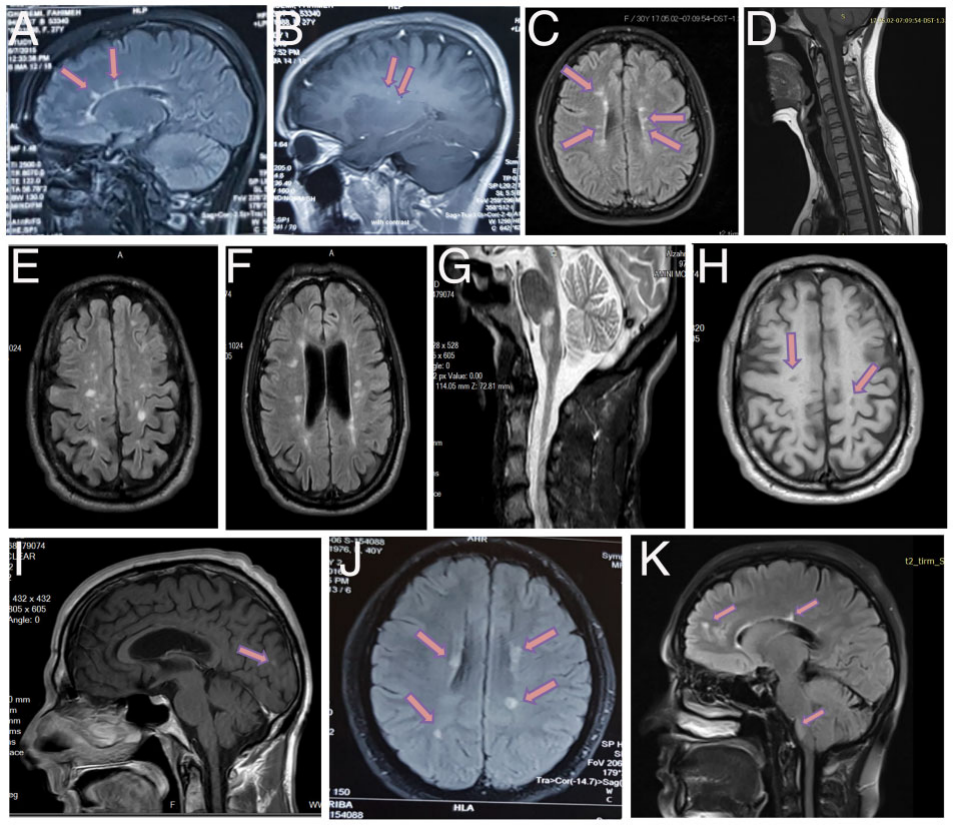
Brain MRI. Axial contrast-enhanced T2W MRI image of the brain of Cases V:6, V:1 and V:3. *Case V:6* (A) Midline sagittal flair (B) and parasagittal T1+GAD images, at 27 years of age, show multiple high-signal foci in the corpus callosum and paraventricular space with enhancing. (C) Axial FLAIR image, at 29 years of age, shows bilateral periventricular high-signal foci. (D) Spinal cord atrophy is shown. *Case V:3* (E, F) Axial FLAIR images, at the age of 39, show bilateral juxta cortical, periventricular and paraventricular high-signal foci and ventricular dilation due to brain atrophy. (G) Sagittal T2 weighted image shows confluent plaques in pons and medulla. (H) Axial T1 weighted image shows some black hole lesions in paraventricular space due to severe axonal injury and loss. (I) Faint enhancement after contrast media administration. *Case V:4* (J) At the age of 42, axial FLAIR image shows right periventricular, and juxta cortical and left paraventricular high-signal foci. (K) Sagittal Flair image, at 44 years of age, shows periventricular and white matter lesions and involvement of pons.
